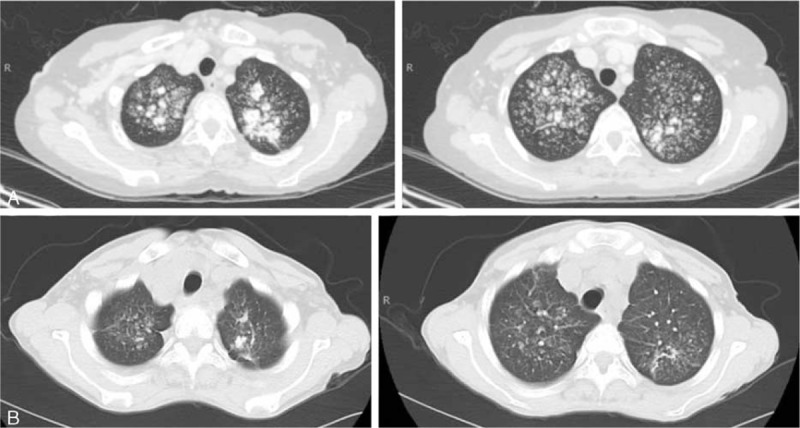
Chest CT scan at first clinical evaluation and 1 year later. A, Native CT examination at diagnosis, showing alveolar calcifications located predominantly in the upper lobes. B, One year later, after 8 consecutive months of sodium thiosulfate and cinacalcet therapy, CT examination revealed a regression of the lung calcifications. CT = computerized tomography
